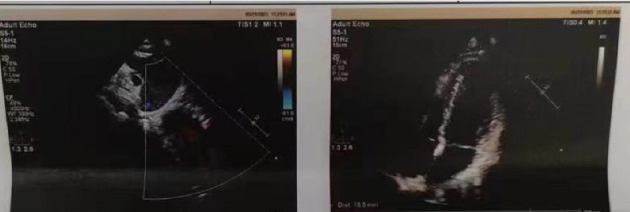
Cardiac ultrasound: no significant abnormalities detected in cardiac structure and blood flow for Case 2.
